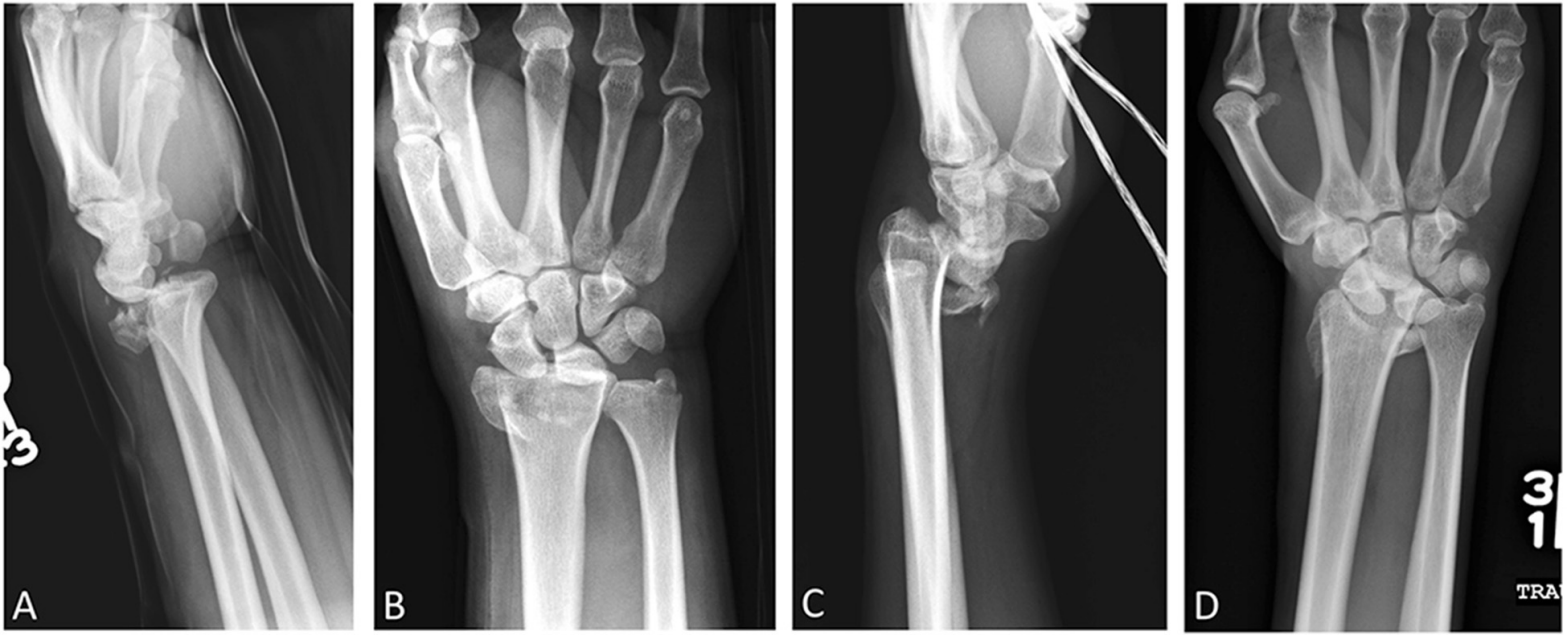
Barton’s and reverse Barton’s. Lateral (a) and AP (b) right wrist radiographs. Comminuted oblique intra-articular fracture with dorsal migration of the carpus, in keeping with Barton’s fracture. c, d Similar fracture morphology but located at the ventral aspect of the radius, with ventral carpal displacement
